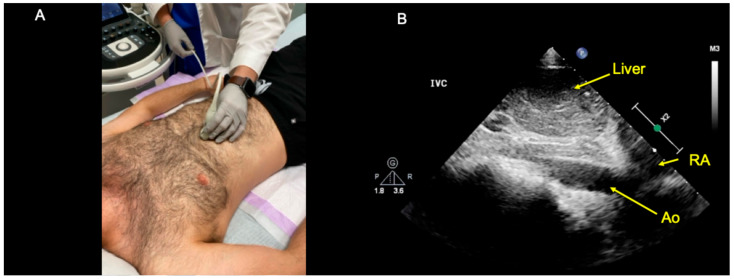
(A) Probe positioning used to obtain the subxiphoid long-axis view of the abdominal aorta. A low-frequency transducer is placed in the subxyphoid region in the parasagittal plane near the patient’s midline, with the probe indicator pointing cranially. The ultrasound beam is then fanned to the patient’s left side slightly until the aorta is visualized. (B) Example of a long-axis view of the abdominal aorta that was misidentified as the IVC by an ultrasound learner (the learner’s image label “IVC” remains included). As shown in this image, in a long-axis view of the abdominal aorta, the cranial portion of the aorta often appears confluent with the right atrium (RA) or other cardiac chambers. See also Supplementary Video S1 for additional examples of subxiphoid abdominal aorta long-axis views that ultrasound trainees have misidentified as the abdominal aorta when using a single criterion to identify the IVC (“the vessel that drains into the RA”).
